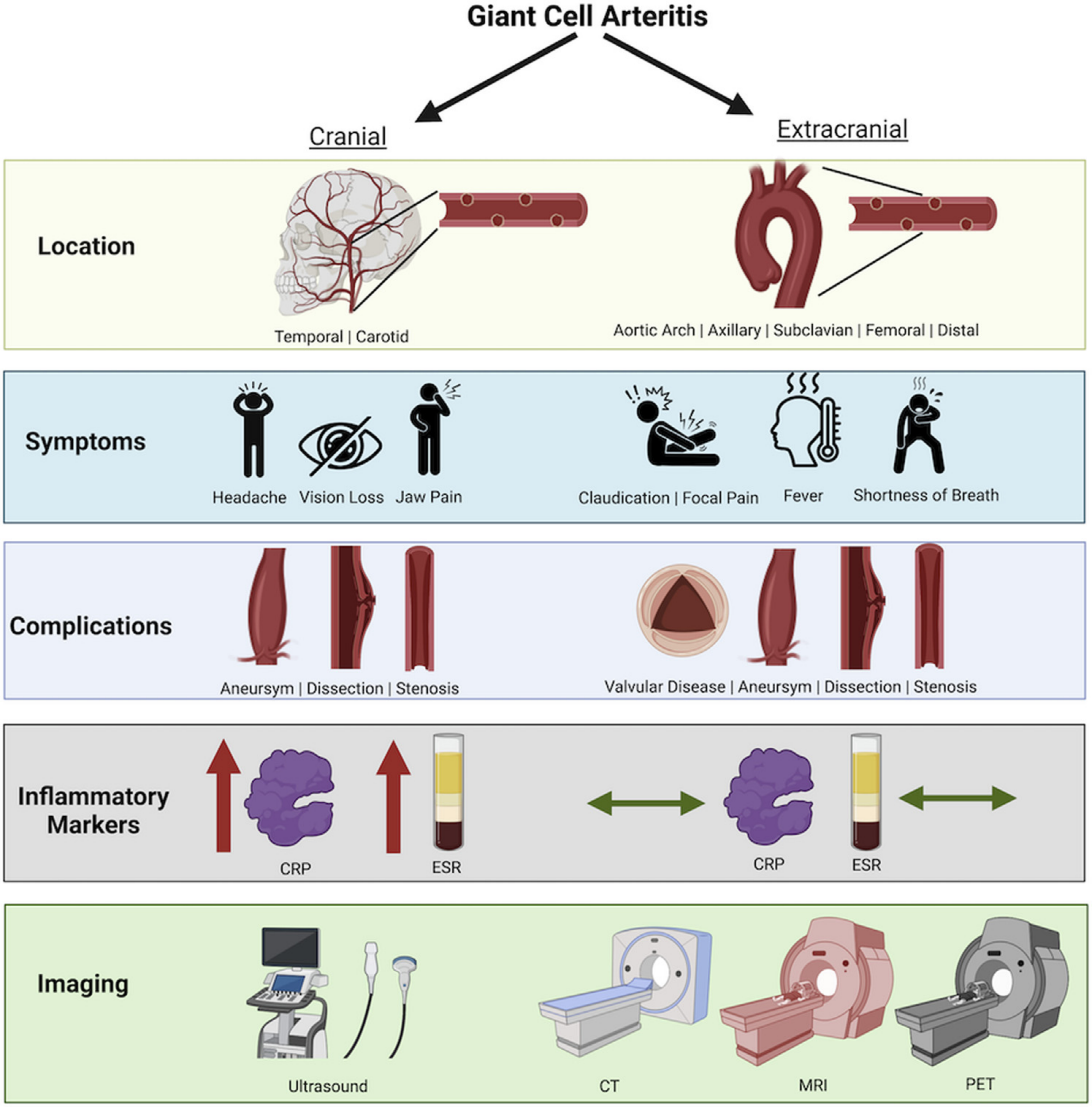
Differences Between Cranial and Extracranial Giant Cell Arteritis Differences between cranial and extracranial giant cell arteritis in anatomical locations, symptoms, complications, laboratory findings and imaging modalities. CRP= C-reactive protein; CT = computed tomography; ESR = erythrocyte sedimentation rate; MRI = magnetic resonance imaging; PET = positron emission tomography.
